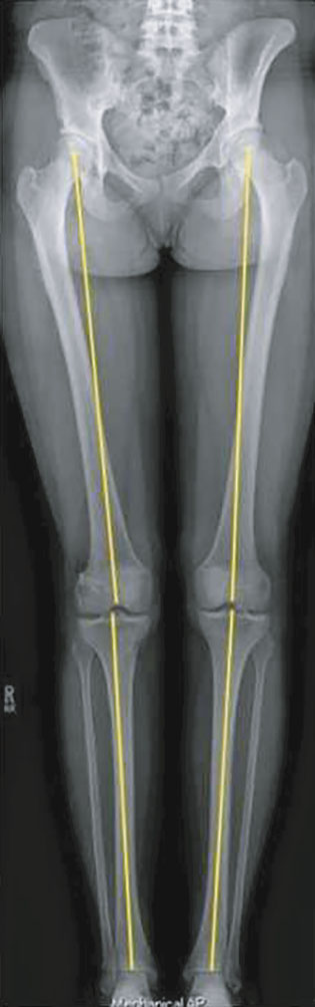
Standing mechanical axis anterior-posterior radiograph at 3 years postoperative.
